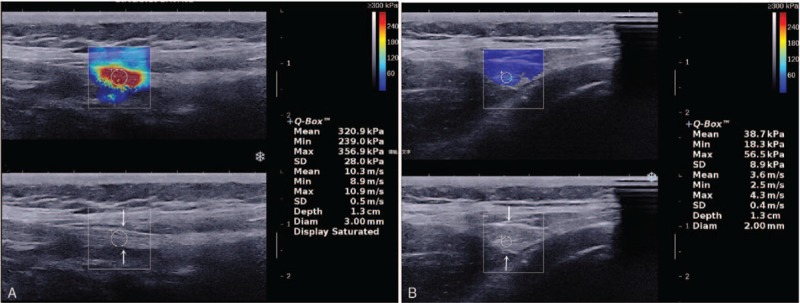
An 8-year-old boy with gluteal muscle contracture. (A) Longitudinal view of the gluteus maximus muscle. The hyperechoic contracture strip can be identified easily (arrows). Its color-coded shear-wave elastogram was mainly orange-red. (B) Transverse view of the gluteus maximus muscle. Gray-scale ultrasound image clearly shows the strip (arrows). The color-coded shear-wave elastogram of the contracture strip was blue.
